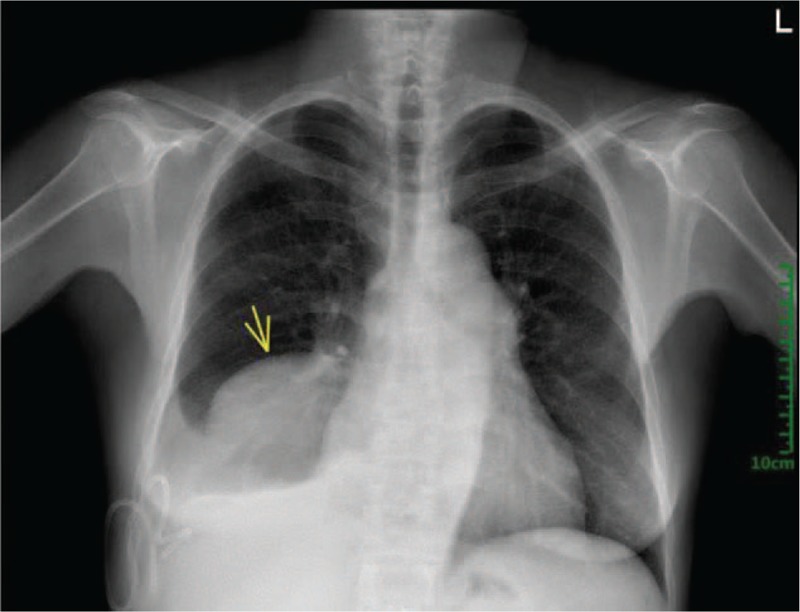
Chest X-ray findings. A large soft tissue density mass (yellow arrow) in the posterior right lower thorax on a posteroanterior chest radiography.
